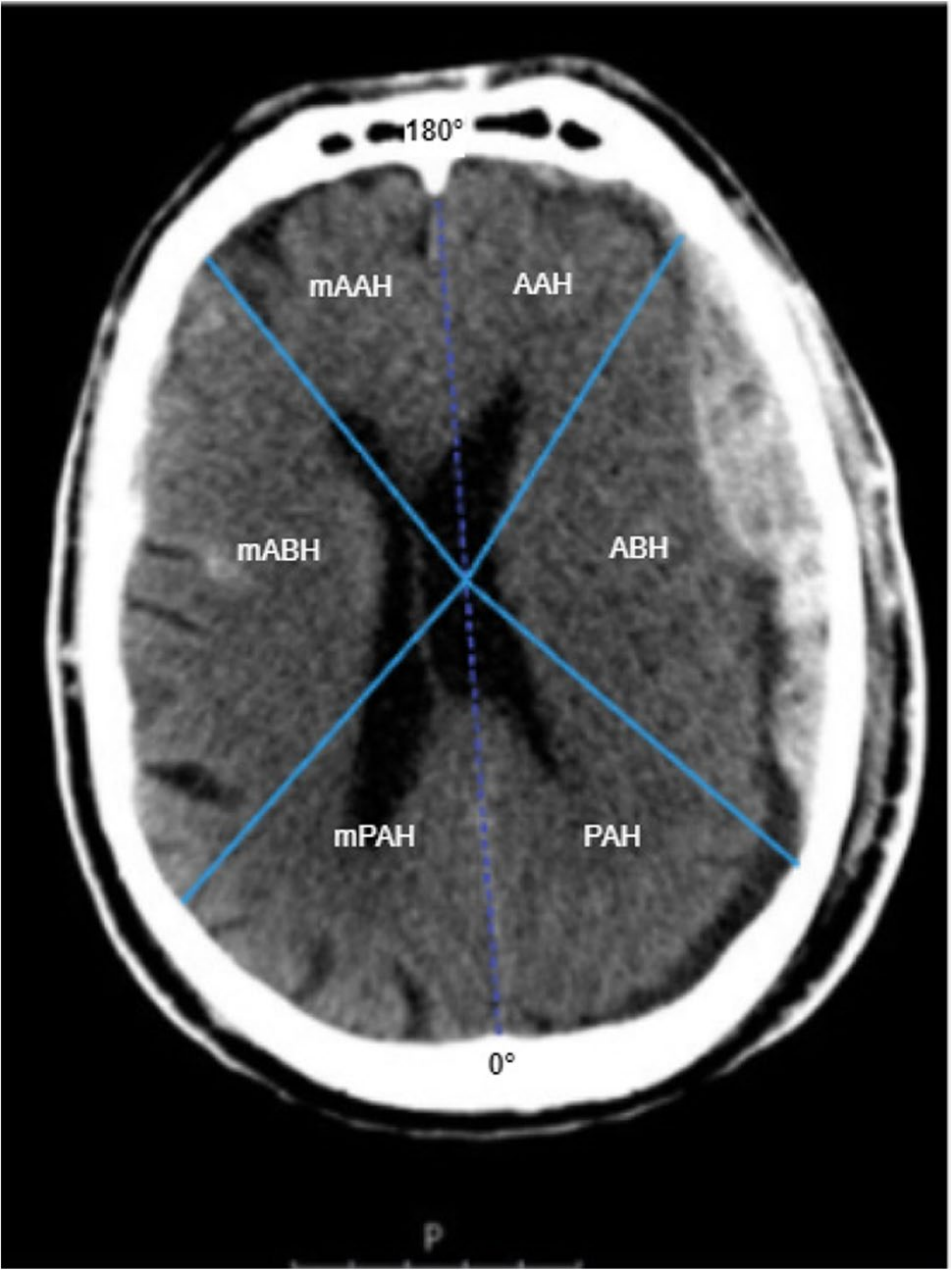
Native CT scan and identification of the analyzed areas
